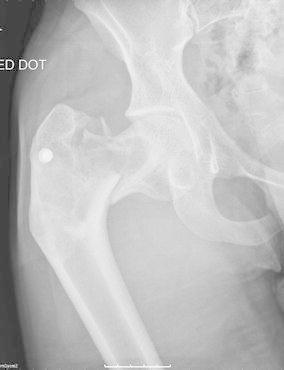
Antero-posterior radiograph of the right hip showing a shepherd’s crook deformity with an intra-capsular femoral neck fracture
